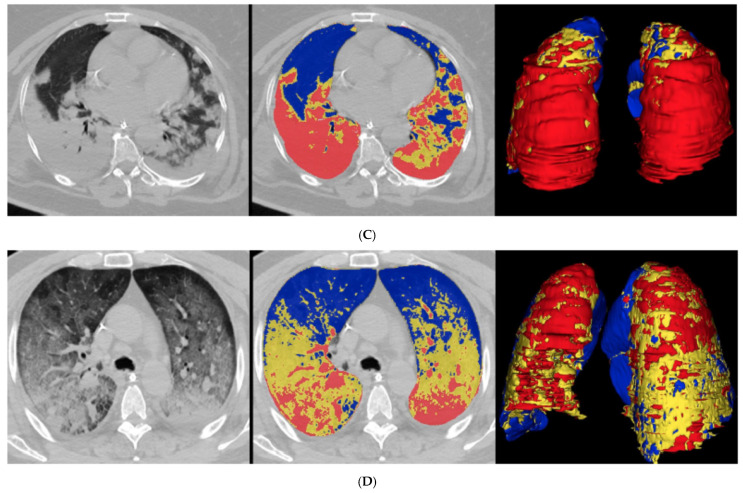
Unenhanced CT scan and QCT analysis in four patients with COVID-19 pneumonia. (A,B) show one QCT with %CL < 23% and one with %CL > 23%. (C,D) highlight the texture differences between the “poorly aerated” volume (%PAL, −500, −100 HU) and “non-aerated” volume (%NNL, 100, -100 HU). (A) refers to a 71-year-old man, oxygenated through nasal cannulae. Upon admission, laboratory tests were as follows: D-dimer 199 ng/mL, fibrinogen 416 mg/dL, PT ratio 1.03, CRP 1.0 mg/dL. %CL was 10%. Non-contrast axial chest CT showed ground-glass opacities, predominantly in the middle lobe; quantitative analysis highlights poorly aerated lung volumes in yellow (8% PAL) and normally aerated lung volumes in blue. (B) refers to a 64-year-old man who required mechanical ventilation and intubation. Upon admission, laboratory tests were as follows: D-dimer 1161 ng/mL, fibrinogen 1021 mg/dL, PT ratio 1.18, CRP 45.3 mg/dL. %CL was 55%. Non-contrast axial chest CT showed diffuse bilateral ground-glass opacities and posterior lung consolidation; quantitative analysis highlights non-aerated lung volumes in red (19% NNL), poorly aerated lung volumes in yellow (36% PAL), and normally aerated lung volumes in blue. (C) refers to a 65-year-old woman who required mechanical ventilation and intubation. Upon admission, laboratory tests were as follows: PT ratio 1.34, CRP 31.9 mg/dL. %CL was 66%. Non-contrast axial chest CT showed extensive posterior consolidation of the right lower lung lobe and patchy dense consolidation in the subpleural space of both left lower and upper lung lobes; quantitative analysis highlights poorly aerated lung volumes in yellow (23% PAL) and non-aerated lung volumes in red (43% NNL). (D) refers to a 66-year-old man who required mechanical ventilation and intubation. Upon admission, laboratory tests were as follows: D-dimer 1335 ng/mL, fibrinogen 832 mg/dL, PT ratio 1.20, CRP 33.8 mg/dL. %CL was 62%. Non-contrast axial chest CT showed extensive ground-glass consolidation with “crazy paving” pattern typical of COVID-19; quantitative analysis highlights normally aerated lung volumes in blue, non-aerated lung volumes in red (12% NNL), and poorly aerated lung volumes in yellow (50% PAL).
